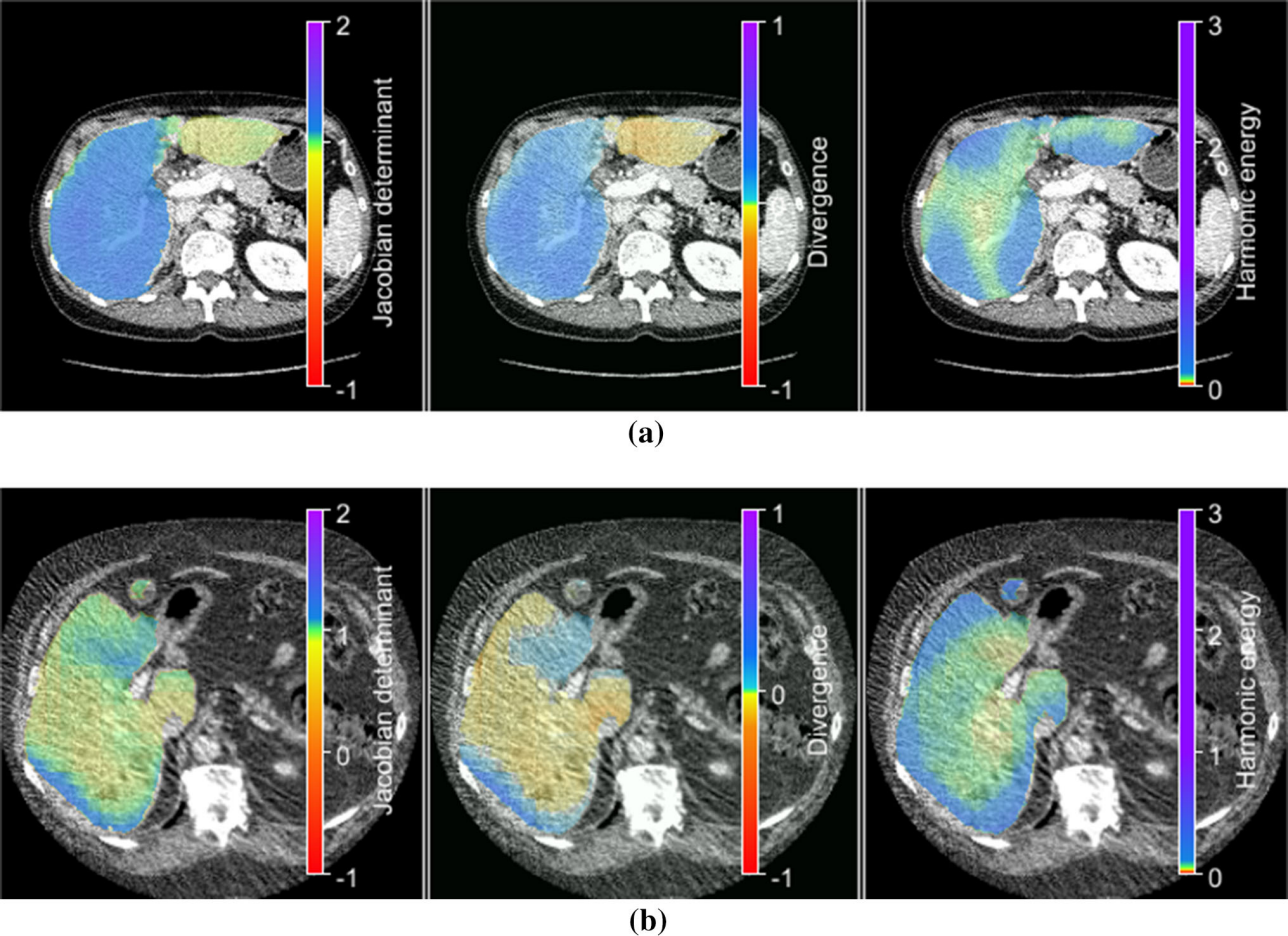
Visualization of the local distributions of the Jacobian determinant (first column), the divergence (second column) and harmonic energy maps (last column) inside the liver as color-overlay on the PV CT images of two patients (a, b). The displayed maps were calculated from the PV CT-CE T1W deformation fields. Range of Jacobian determinant, mean absolute divergence, and harmonic energy are given by the color, respectively. a$$J\in (0.54,1.57),\hbox { mean}(|\hbox {div(y)}|)=0.09,\hbox { HE}=0.09$$. b$$J\in (0.49,1.91),\hbox { mean}(|\hbox {div(y)}|)=0.15,\hbox { HE}=0.14$$
